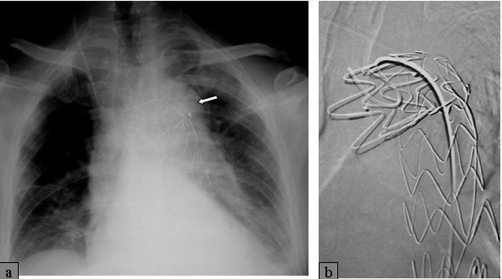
Chest X-ray two weeks after successful aorta stent deployment.
